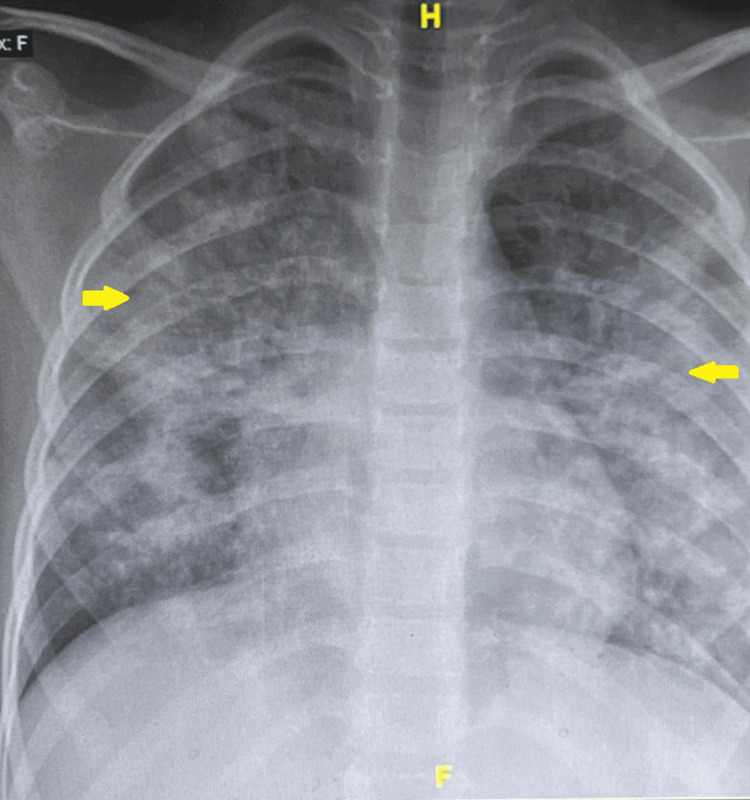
Chest X-ray at the time of admission. Chest radiography demonstrated bilateral non‑homogeneous opacities involving the middle and lower lung zones, as demonstrated by the yellow arrow.
